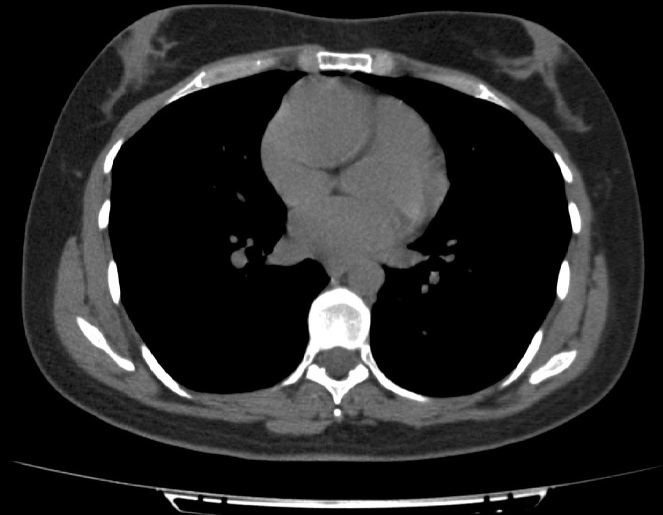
CT lung scan: isodence mass with no calcifications determining bulge on the right antero lateral cardiac border.
